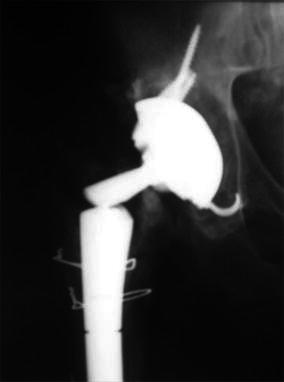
Radiograph shows dissociation of the femoral neck–stem interface
